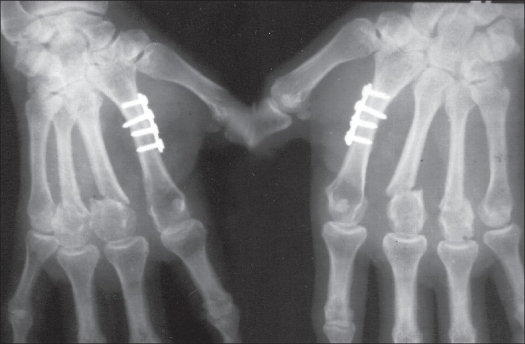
Radiographs showing union of fractures after removal of Kirschner wires
